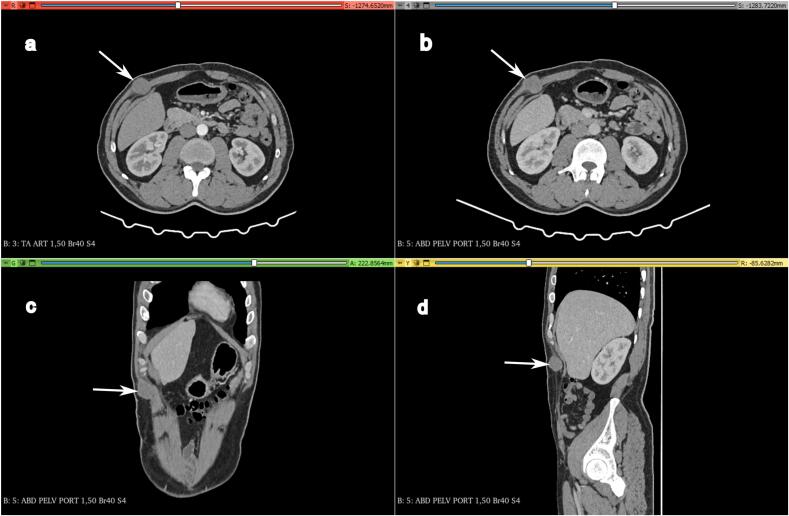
CT imaging showing the tumor (a) Arterial phase axial view and (b) portal phase axial view CT images showing no specific pattern of enhancement of the tumor (White arrow) (c) Coronal view and (d) sagittal view CT images revealing a 3 cm mass (White arrow) in the aponeurosis of the right external oblique muscle with well-defined contours.
